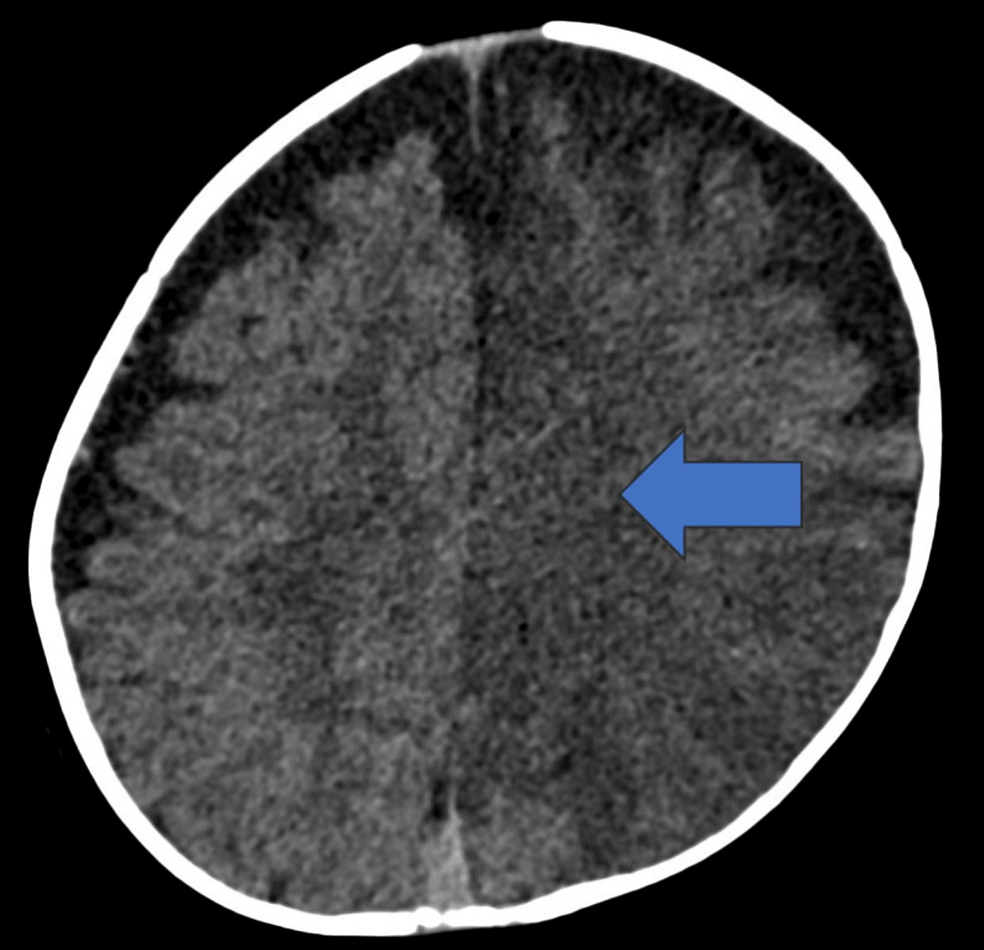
Axial non-contrast CT of the head demonstrates a large area of hypoattenuation and loss of gray-white matter differentiation in the left cerebral hemisphere. The blue arrow shows a large area of hypoattenuation and loss of gray-white matter differentiation in the left cerebral hemisphere.
